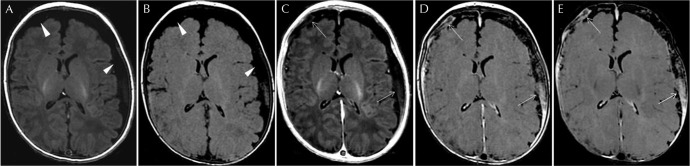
Synthetic MRI. (A) T_1_IR and (B) FLAIR show subdural effusion (arrowheads). CE (C) T_1_IR shows subtle enhancements (arrows). CE (D) FLAIR and (E) DIR show a clear enhancement in the subdural effusion area (arrows). MRI, magnetic resonance imaging; T_1_IR, T_1_ weighted inversion recovery; DIR, double IR; CE, Contrast-enhanced.
